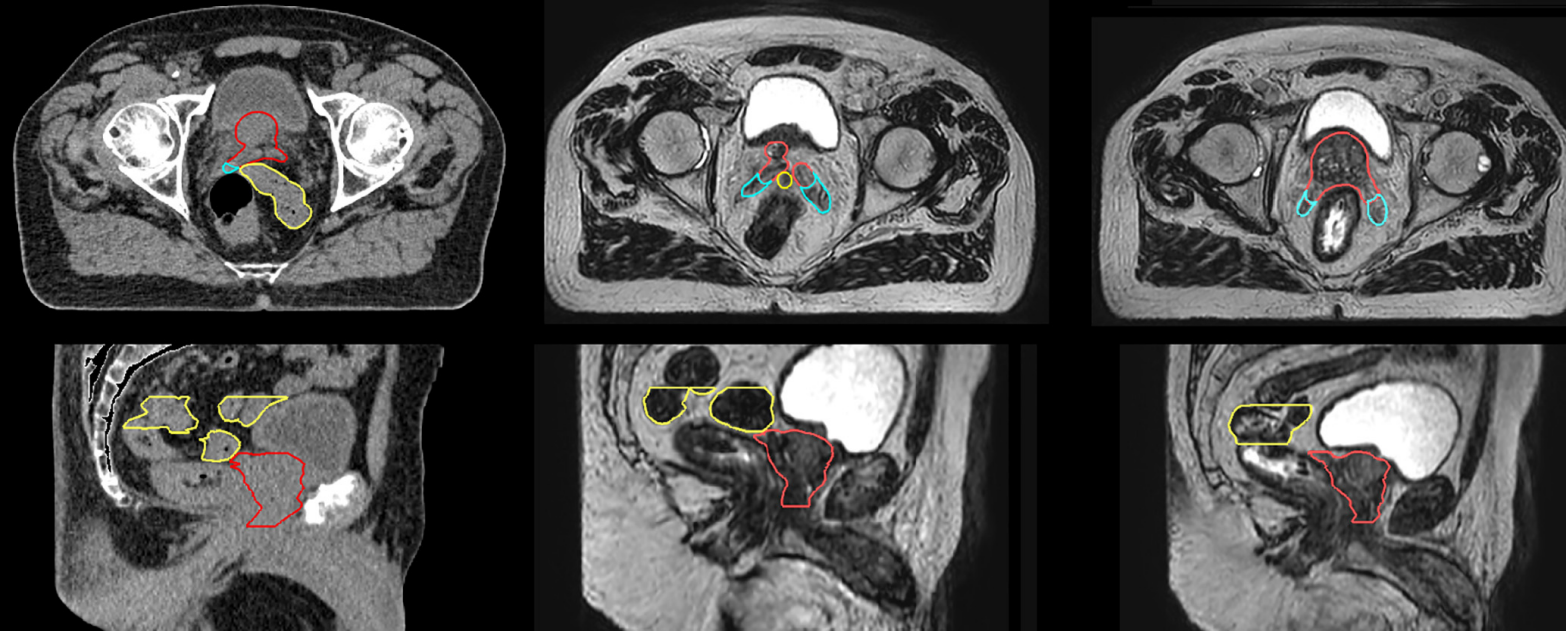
Axial (top row) and sagittal (bottom row) images of case 3 from the planning CT (left column), #5 verification MR acquisition (centre column), and #6 verification MR acquisition (right column). In all images, the prostate CTV (including proximal 1 cm seminal vesicles), SV CTV, and bowel are shown as red, cyan, and yellow contours, respectively. (For interpretation of the references to colour in this figure legend, the reader is referred to the web version of this article.)
